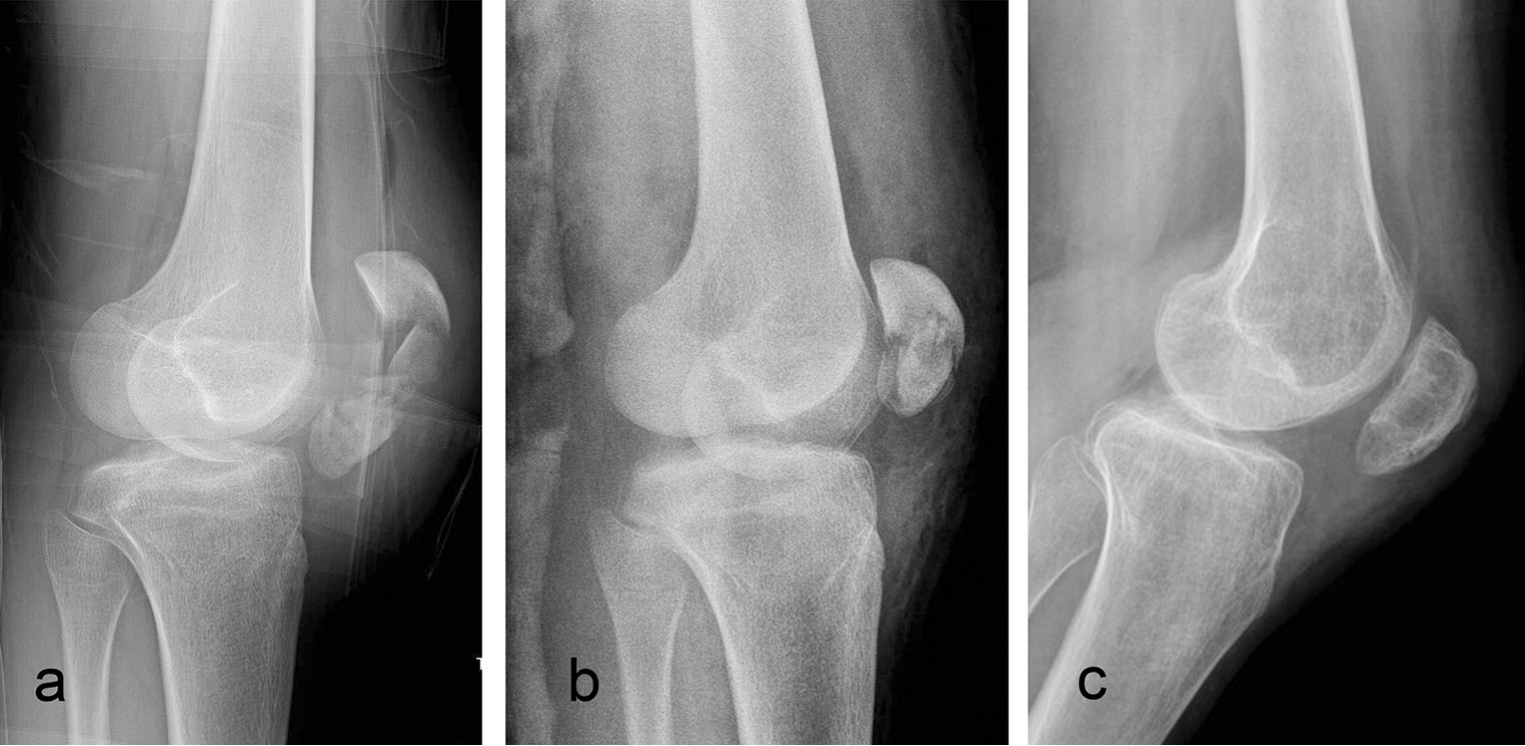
Comminuted patellar fracture. Preoperative X-ray (a); postoperative X-ray (b); final follow-up X-ray (c)
